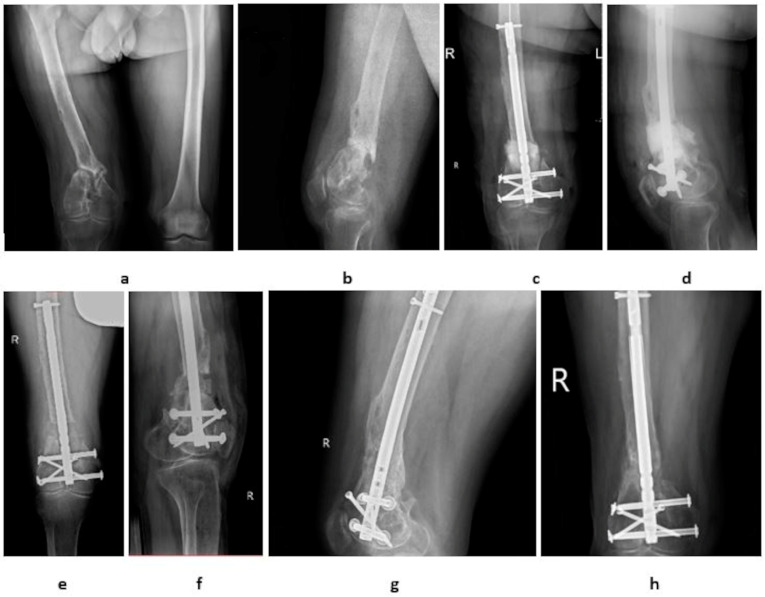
A 37-year-old man with post-traumatic pseudoarthritis of the right femur (4 years after injury). (a,b) preoperative femoral pseudoarthritis; (c,d) first-stage IMT postoperative X-ray, resection of pseudoarthritis, IMN and PMMA (with clindamycin, gentamycin, vancomycin); (e,f) second-stage IMT postoperative X-ray, removal of PMMA, autograft of iliac crest bone grafting; (g,h) healthy bore union of new distal femur.
